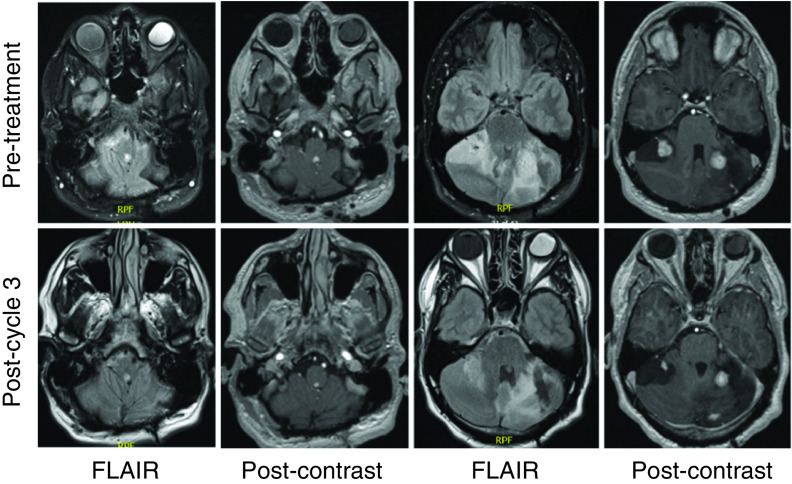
Pre- and post-treatment MRI imaging for patient 2. The time interval between scans was 4 months, and the patient had received three cycles of belzutifan. Panels show fourth ventricular mass and multiple cerebellar hemangioblastomas, with interval reduction in perilesional edema and reduced contrast enhancement. FLAIR: Fluid-attenuated inversion recovery.
